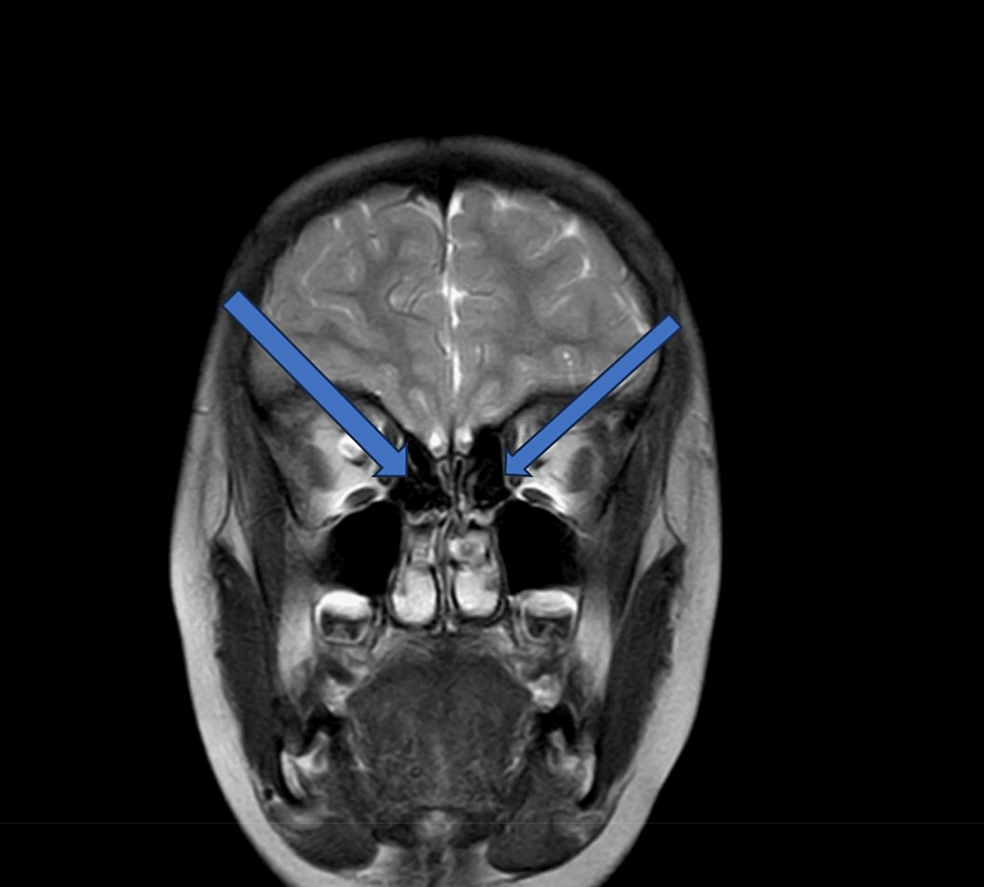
MRI image depicting findings suggestive of an acquired demyelinating disorder in the brain
